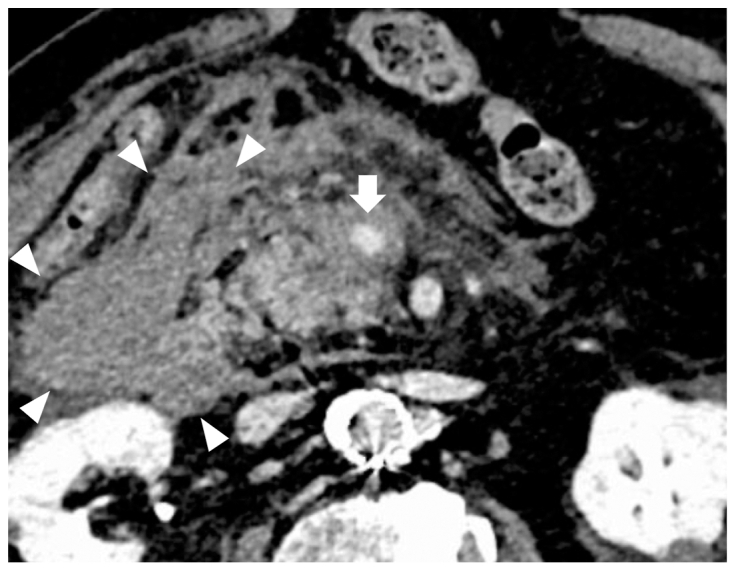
Emergency computed tomography (CT) scan revealing a large hematoma at the retroperitoneum (*white arrowhead*) and a pseudoaneurysm (*white arrow*) on the anterior surface of the pancreatic head.
